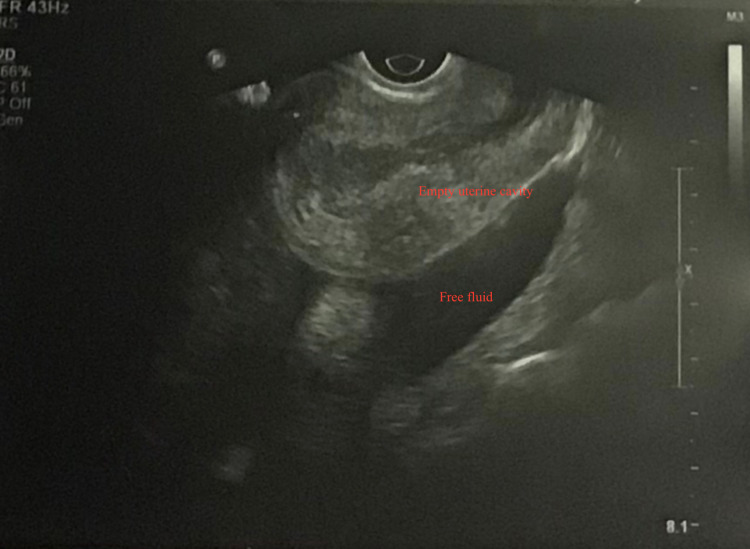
Ultrasound image showing gross hemoperitoneum with the empty uterine cavity
